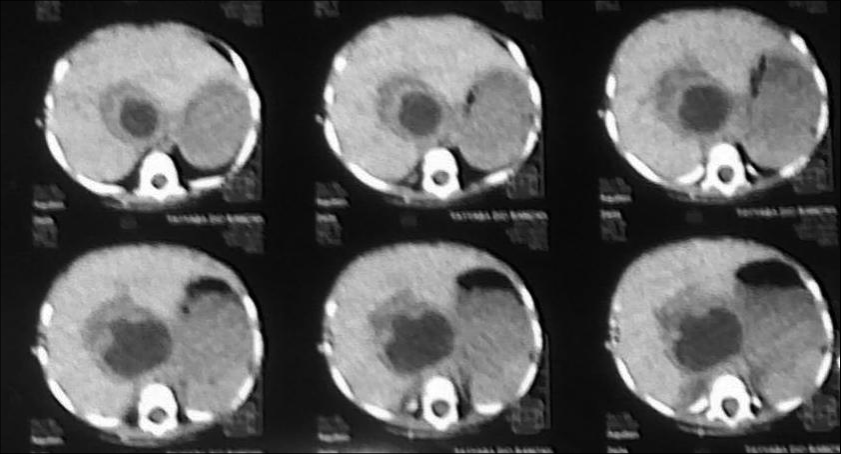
Figure 1: CT scan showing a hypo-dense area at porta hepatis.
